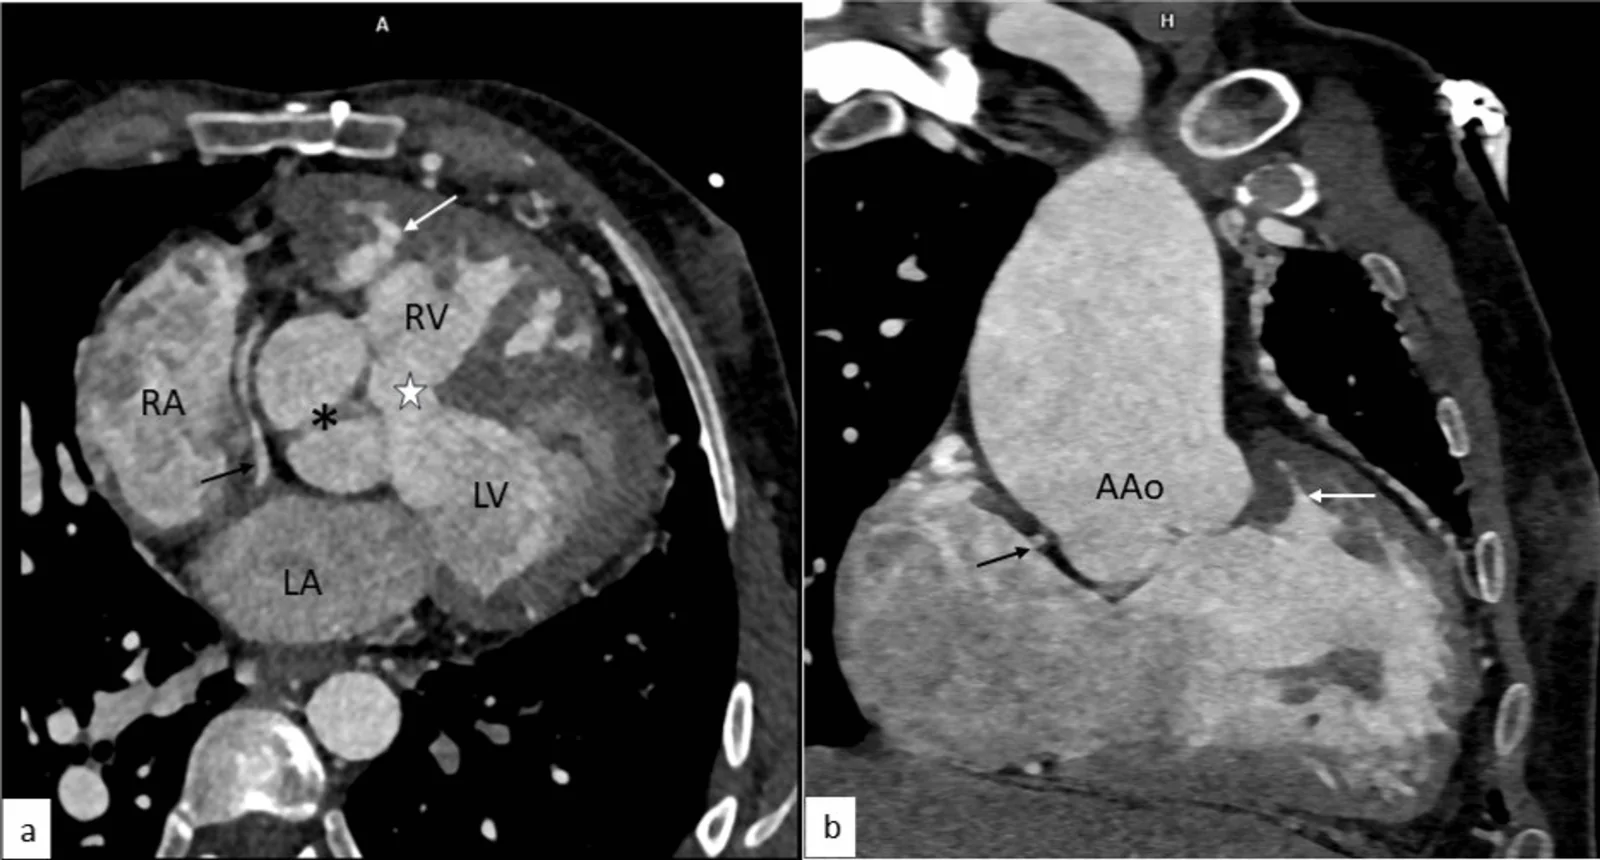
Cardiac CT demonstrating TOF morphology (a) Multislice Cardiac CT: (a) Axial 4- chamber view in diastole, showing subaortic VSD (star) with overriding of the aortic root (asterisk), right ventricular hypertrophy (white arrow) with crowding of papillary muscles. The RA appears dilated. There is a malignant course of the right coronary artery (RCA) between the RA and the aortic root (black arrow); (b) Multislice Cardiac CT: (b) Coronal image focusing on ventricular outflow tract showing marked infundibular stenosis (white arrow) and pulmonary atresia and overriding of the ascending aorta (AAo). Note is made of the RCA between the RA and the aortic root (black arrow). TOF-Tetralogy of Fallot, AAo-Ascending aorta, RA-Right atrium, RV- Right ventricle, LA-Left atrium, LV-Left ventricle, VSD-Ventricular septal defect.
